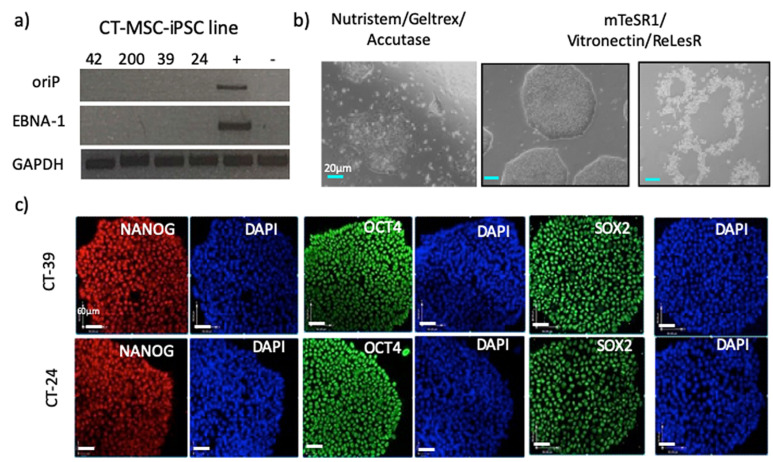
Vector-free pluripotency cell lines established from CT-MSC. (a) The CT-induced pluripotent stem cell (iPSC) lines are negative for the OriP and EBNA genes of the episomes carrying the reprogramming factors by passage 10, indicating the loss of the episome after reprogramming has completed. The positive control (+) is an earlier passage 3 of the CT-42 iPSCs lines still containing the reprogramming vectors, and the negative control (−) is a human embryonic stem cell (ESC) line (CA1). (b) Phase contrast images of the morphology of CT-MSC iPSCs grown in Nutristem on Geltrex matrix compared to mTeSR on Vitronectin matrix. Distinct, uniform colonies with defined borders are observed only with mTeSR1 medium with Vitronectin-coated plates. The iPSC colony can be easily lifted from the plate using ReLeSR leaving behind the differentiated cells that sometimes form at the outer edges of pluripotent stem cell colonies. Scale bar = 20 µm for all panels. (c) Vector-free CT-MSC-iPSC lines express pluripotency markers Nanog, Oct4 and Sox2 as detected by immunocytochemistry. Scale bar = 60 µm for all panels.
